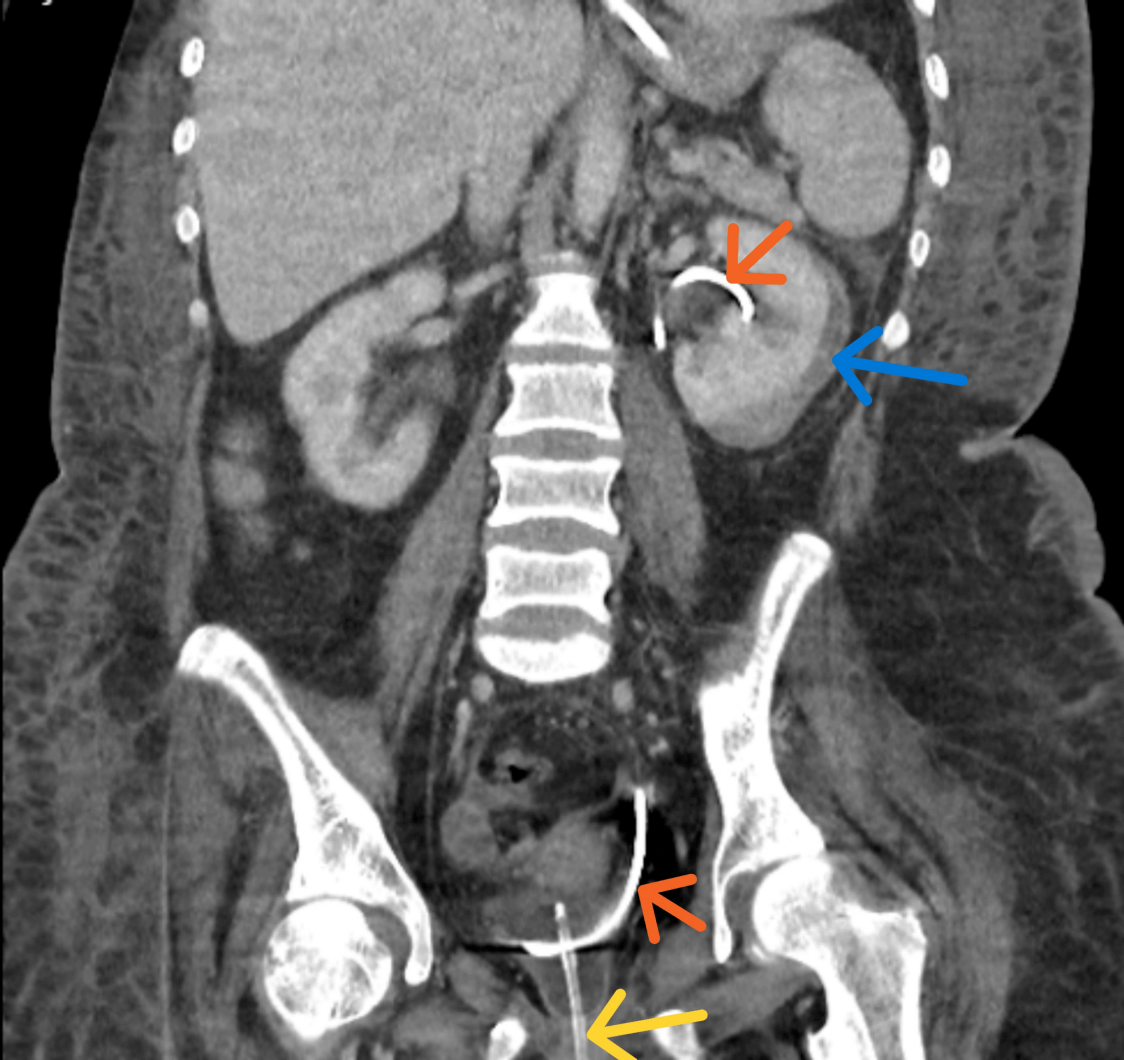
CT of the urinary tract showing a left-sided ureteric stent in situ (orange arrow) and urinary catheter in situ (yellow arrow). Improvement in the left retroperitoneal, pelvic, and abdominal collection was noted. No new collections were identified. Stable appearances of the left kidney subcapsular collection were noted (blue arrow).
